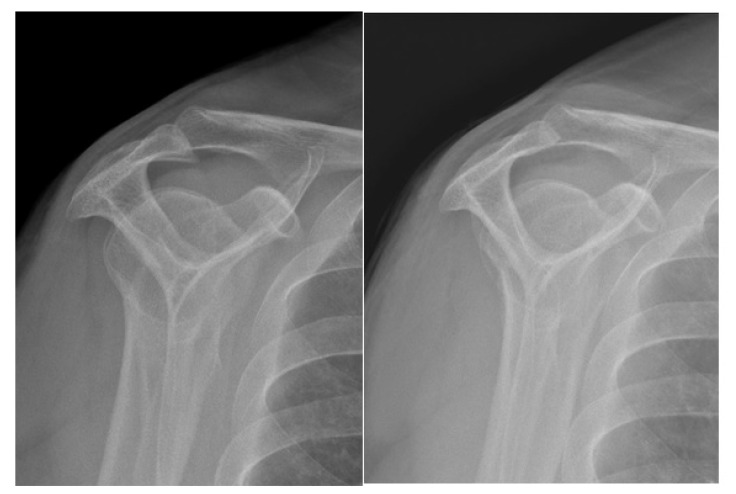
Supraspinatus outlet view of left shoulder showing type III acromion with large subacromial spur and x-ray after subacromial decompression.
